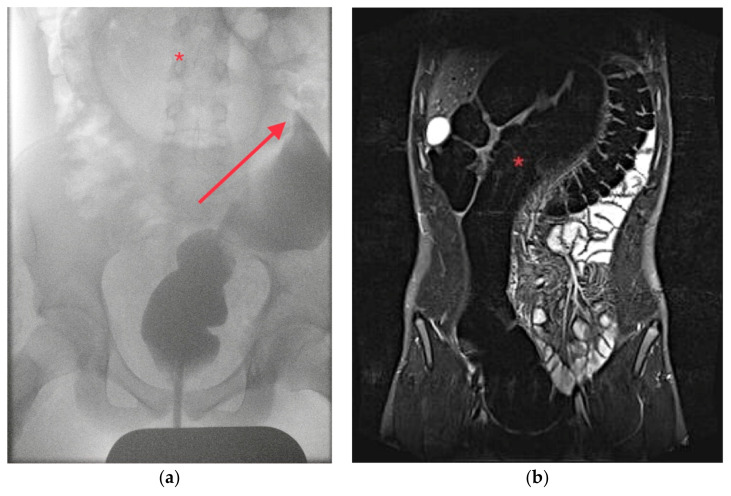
Patient 2: (a) Contrast enema showing the air-filled, dilated sigmoid (*) and the ‘bird’s beak-sign’ (arrow); (b) MRI showing a grossly enlarged and dilated sigmoid (*).
